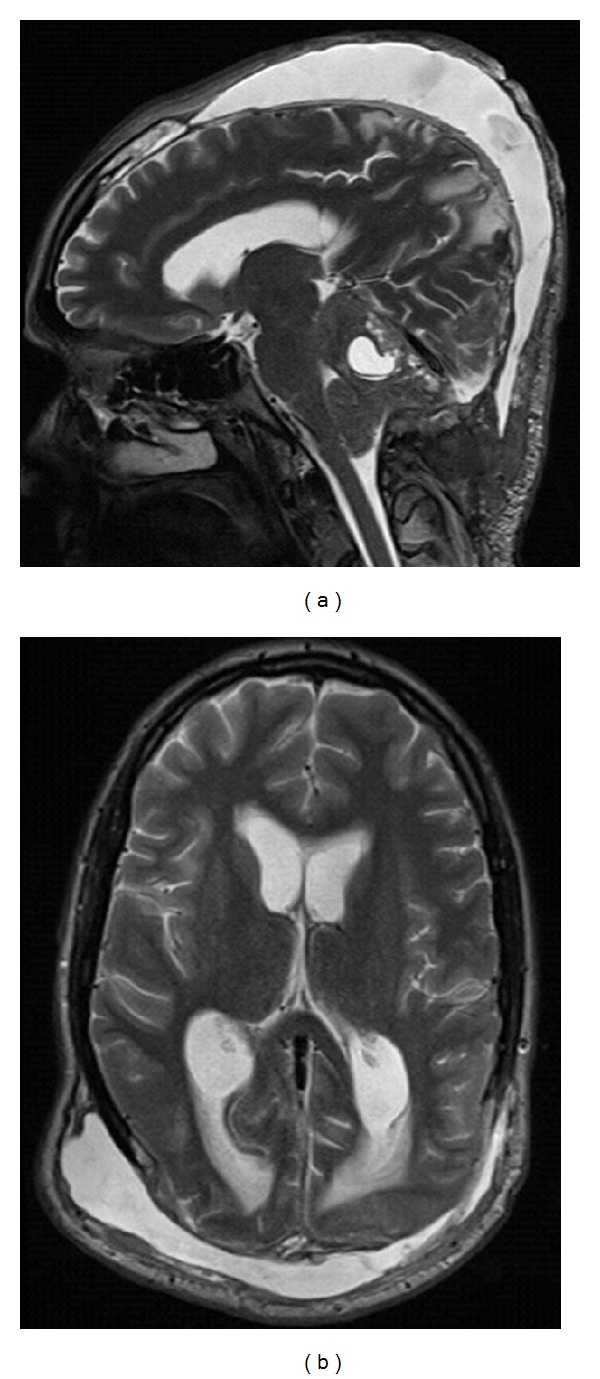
Cranial T2-wighted MRI. (a) Sagittal. (b) Axial. The cranial MRI shows a CSF circulatory disturbance with subcutaneous CSF accumulation.
